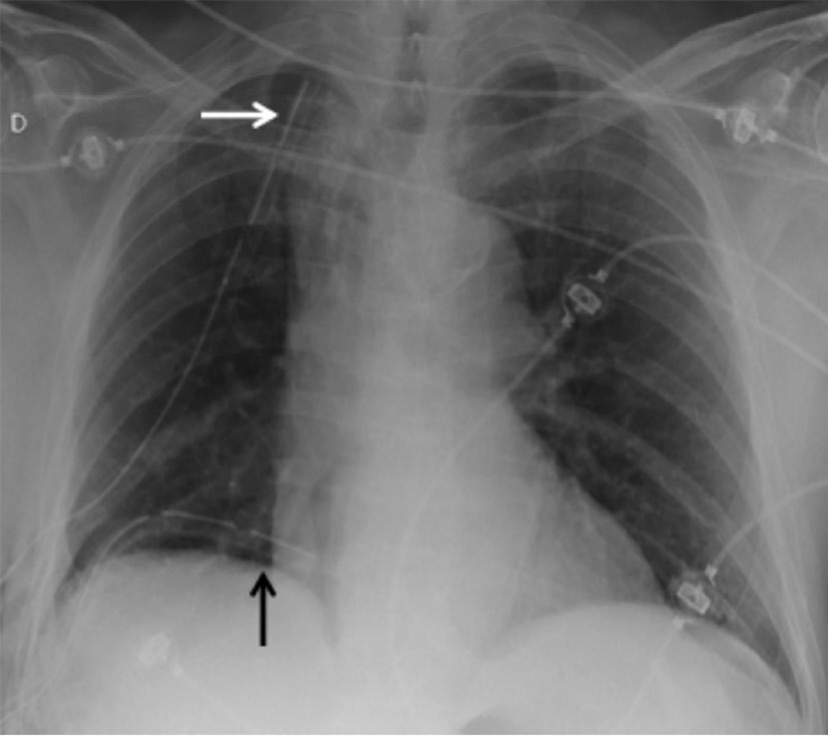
Post-operative radiograph of a right lower lobe resection. CXR allows visualisation of the drains in postero-basal (black arrow) and antero-apical (white arrow) positionsThe early post-operative aspect consists in a pneumothorax or hydropneumothorax of low abundance, parietal emphysema and retraction of the hemithorax. After a few days and weeks, a decrease in the signs of retraction and an expansion of the remaining lobe(s) appear. Later on, imaging shows retraction of the operated hemithorax, mediastinal attraction, filling of the costo-diaphragmatic recess, elevation of the diaphragmatic dome, hilar displacement and pulmonary hyperlucency (related to compensatory hyperinflation with relative vascular rarefaction); parietal sequelae and surgical material persist. Chest X-ray can demonstrate juxta-phrenic peak sign or a pseudo-lobar collapse aspect that can be misleading for atelectasis if the operating history is not known by the radiologist (Fig. 3) [17].
